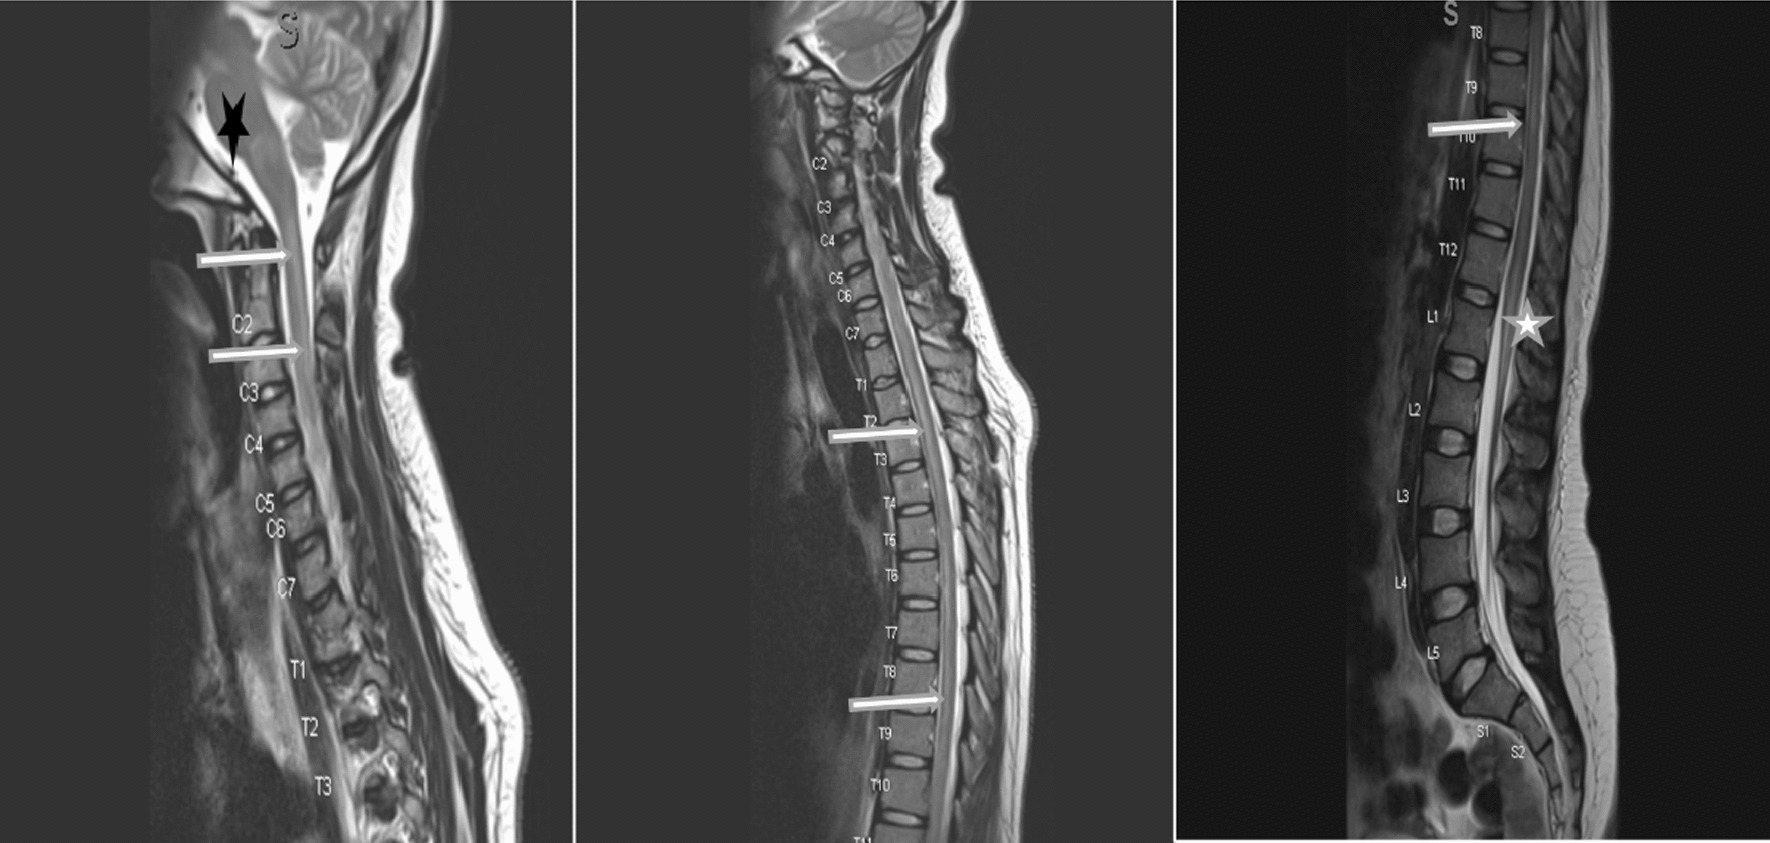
Whole spine magnetic resonance imaging of case 2. T2 weighted images showing hyperintensity of the whole spinal cord (white horizontal arrows), with involvement of the brainstem (black star) and conus medularis (white star)
